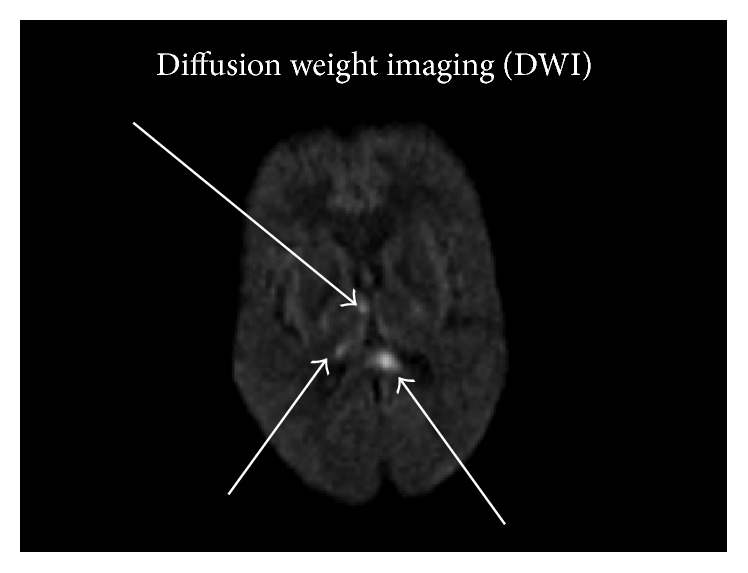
DWI shows bright areas (restricted diffusion) in the splenium of the corpus callosum and right thalamus compatible with infarcts. These areas were low on the apparent diffusion coefficient maps which confirms that these are infarcts (not shown). Infarcts are a known complication of neuro-TB.
